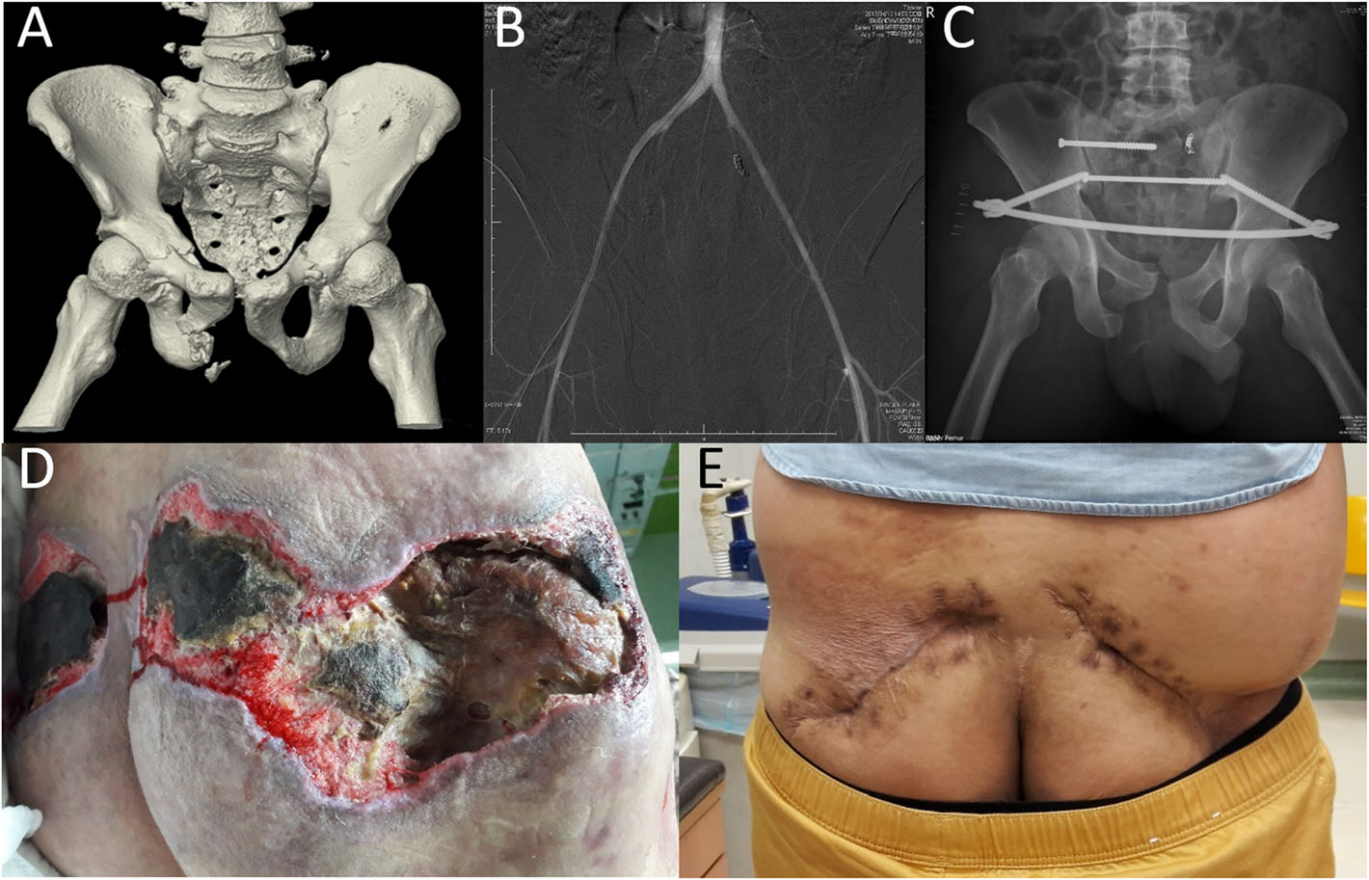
A case with surgical site infection following trans-arterial embolization and osteosynthesis for pelvic ring injury. a Computed tomography revealed an AO/OTA B3.1 pelvic ring injury. b Bilateral internal iliac artery trans-arterial embolization was performed using gelfoam (right side) and metal coil (left side). c Radiographic findings after the osteosynthesis. d Significant bilateral gluteus maximus necrosis was observed at 1 month after the trans-arterial embolization. e Final appearance after treatment for the soft-tissue defect
